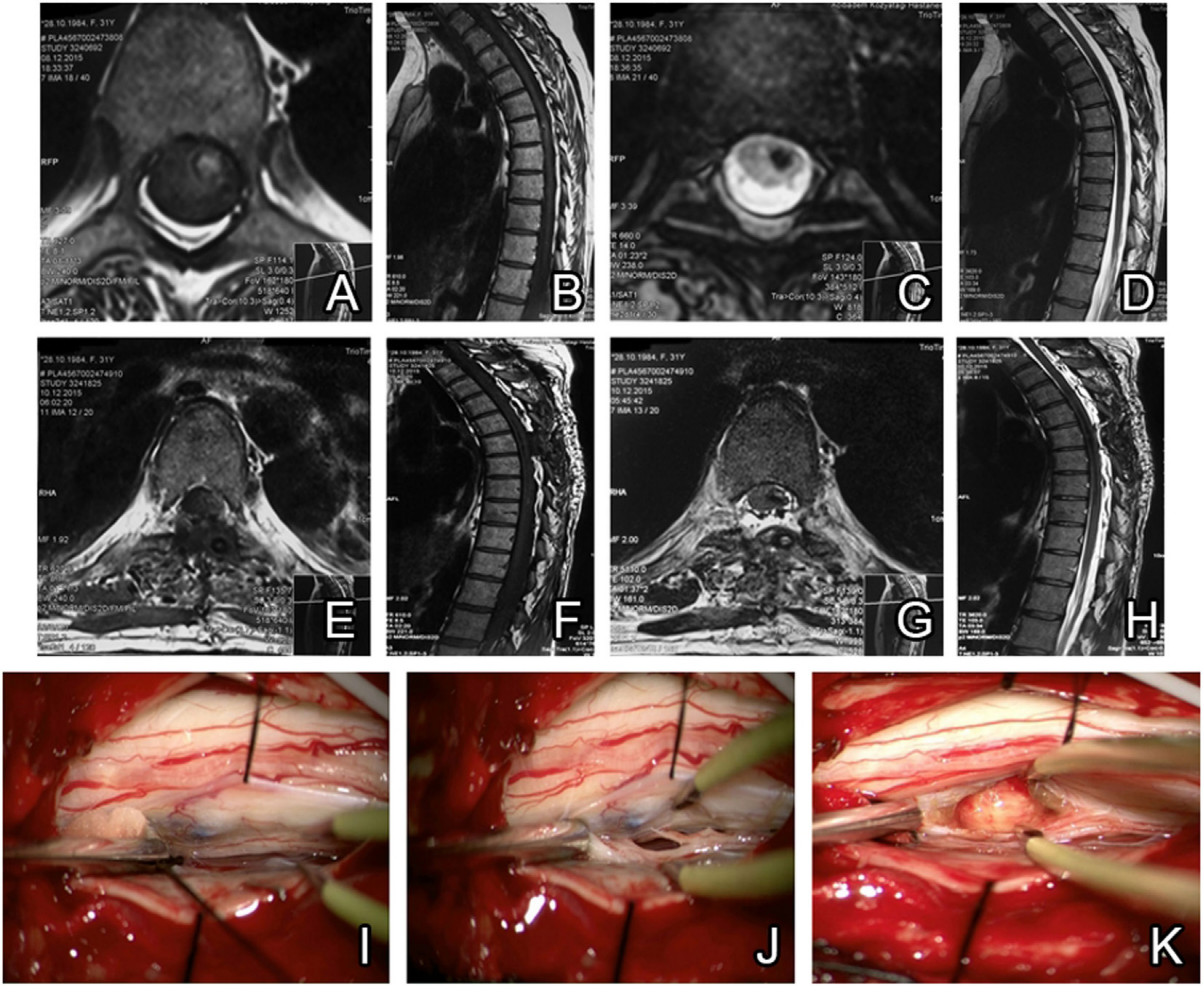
Preoperative MRI scans reveal a thoracal intramedullary located cavernoma. T1 axial and sagittal sections showed hyperintense view of cavernoma (A,B), but hypointense view of cavernoma seen in T2 axial and sagittal sections (C,D). Postoperative MRI confirms total resection without hematoma residual cavernoma (E–H). Intraoperative view of cavernoma before resection (I,J). Gross total resection is confirmed after removal (K).
